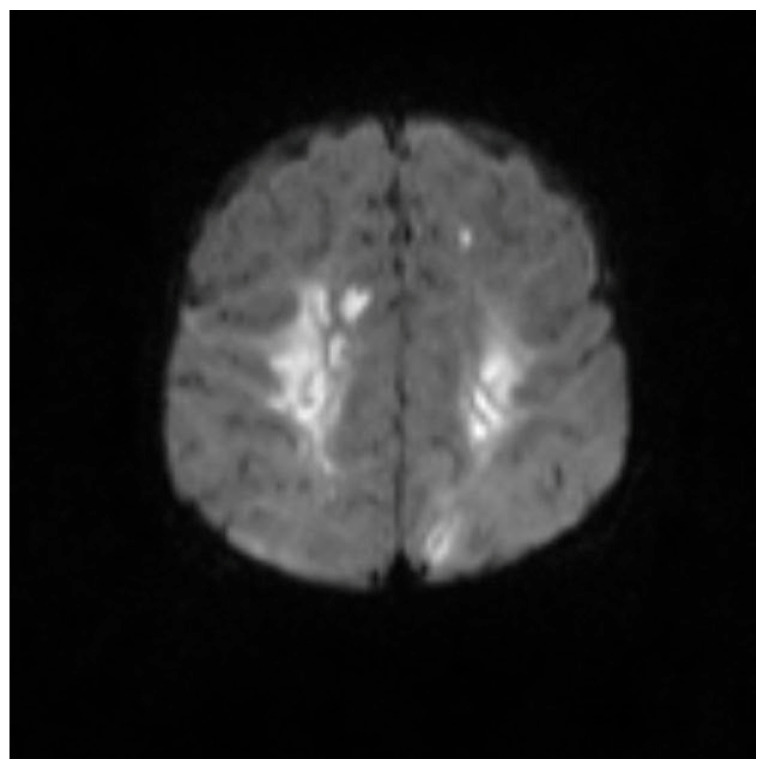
MRI brain scan showing the watershed area infarction.
